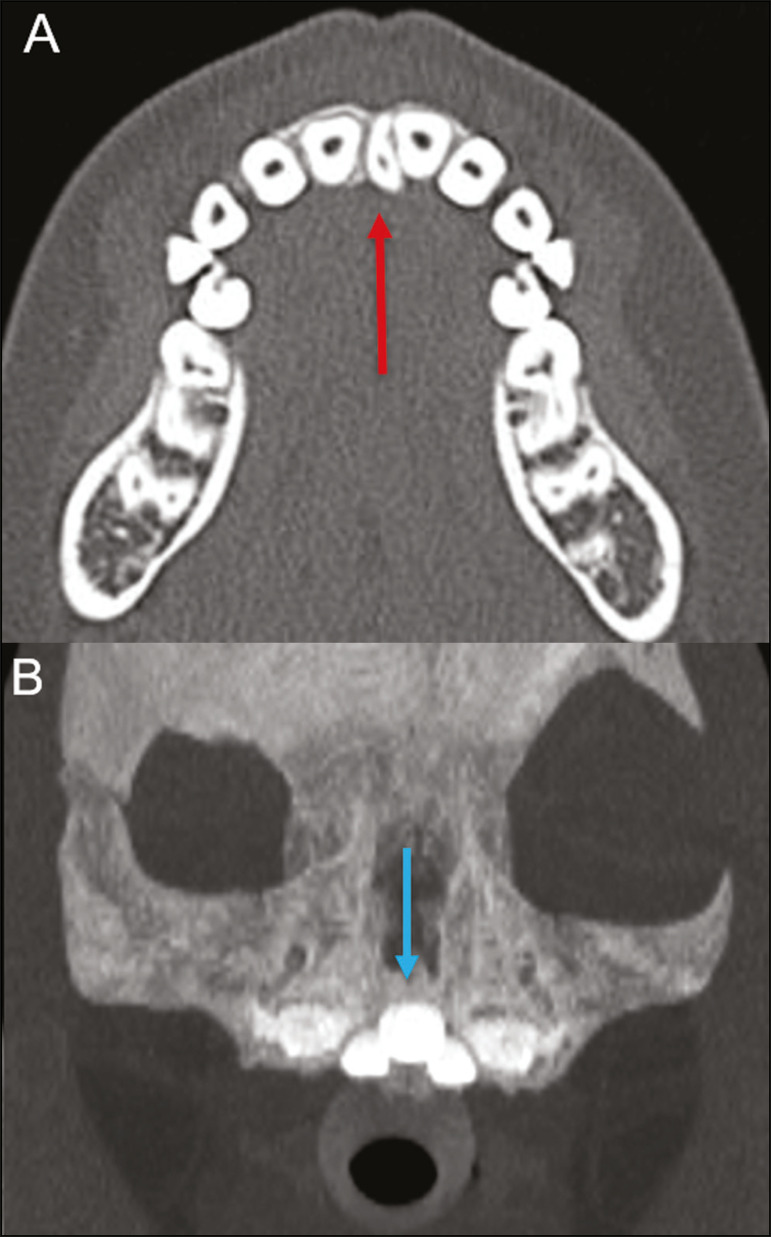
Axial (A) and coronal in maximum intensity projection (B) CT scans showing dental supernumerary element impacted in the midline of the maxila between two central incisors-a *mesiodens* (red arrow)-which differs from the single central incisor (blue arrow).
